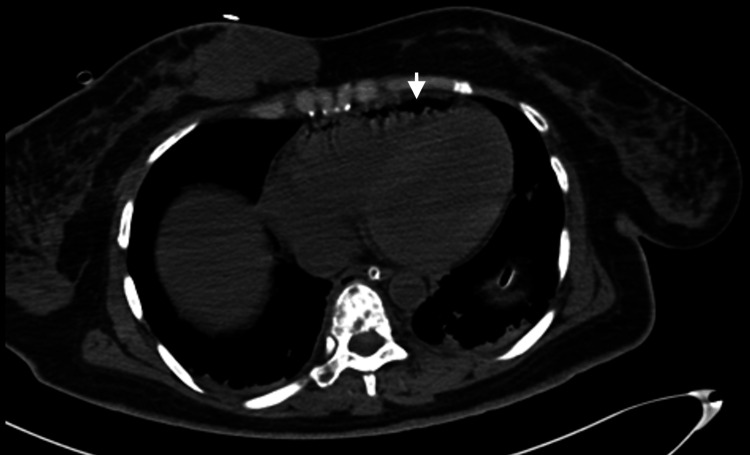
Axial CT scan of the chest with intracardiac gas (white arrow).
